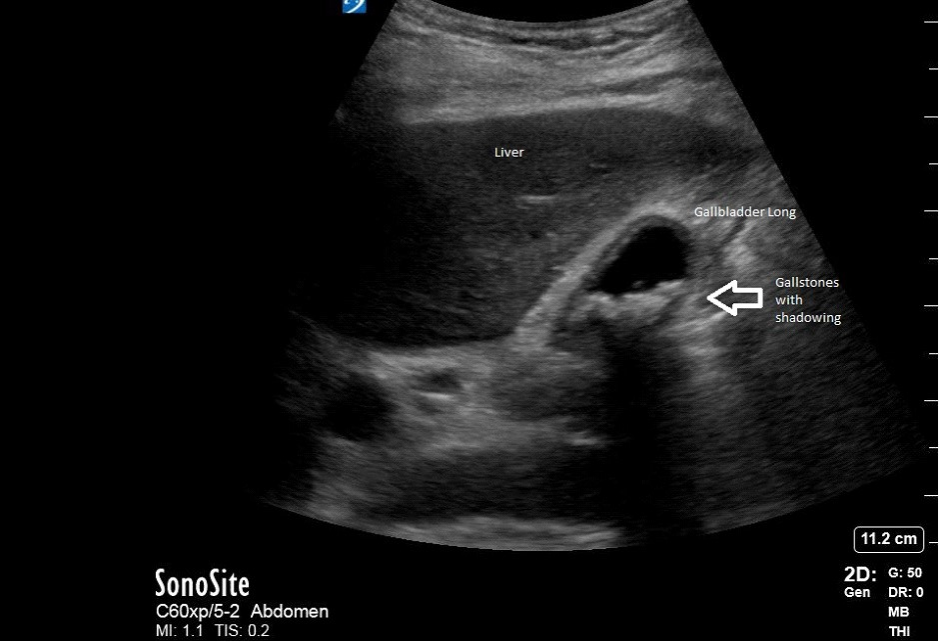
Gallbladder POCUS concerning for acute cholecystitis: gallbladder with thickened anterior wall, shadowing gallstones, and a positive sonographic Murphy sign.
